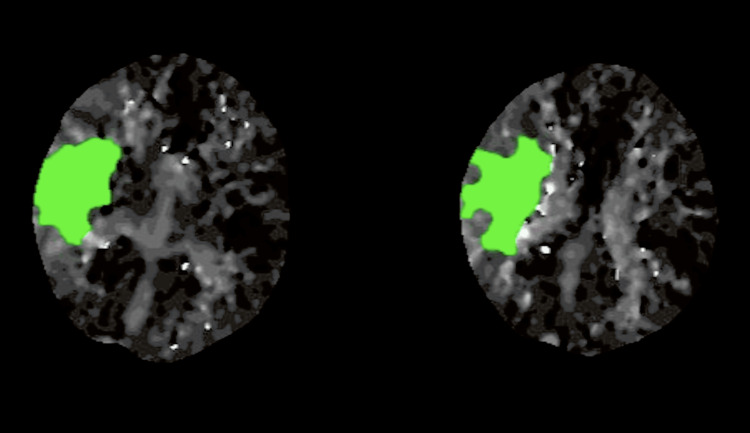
CT perfusion imaging of the brain. CT, computed tomography
